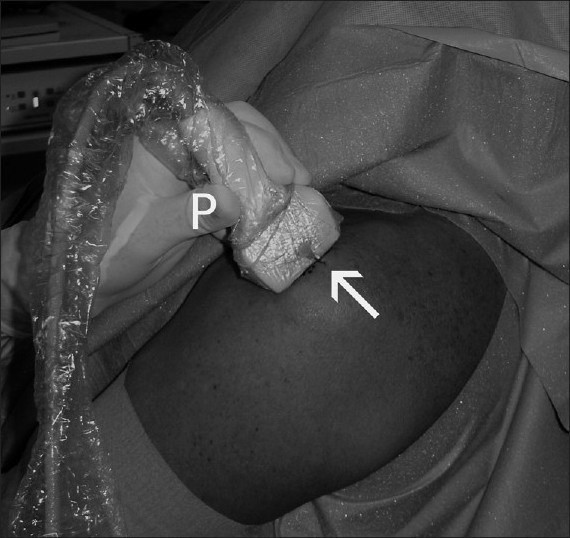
Intraoperative use of ultrasound is shown for needle localization (arrow) of a calcific deposit. A sterile cover is used with a standard superficial probe (P) and sterile ultrasound gel
